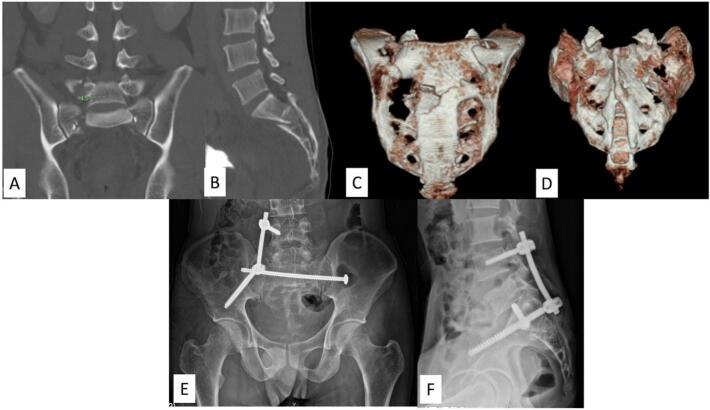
Demonstrating an AO type C3 sacral fracture with intact L5S1 facet joints (case No. 17). (A-D) Preoperative CT scan and CT reconstruction images showing a U-type fracture with a comminution fracture of the right sacral ala and right L5 pedicle. (E, F) Postoperative radiographs after transiliac-transsacral screw fixation with right triangular fixation.
